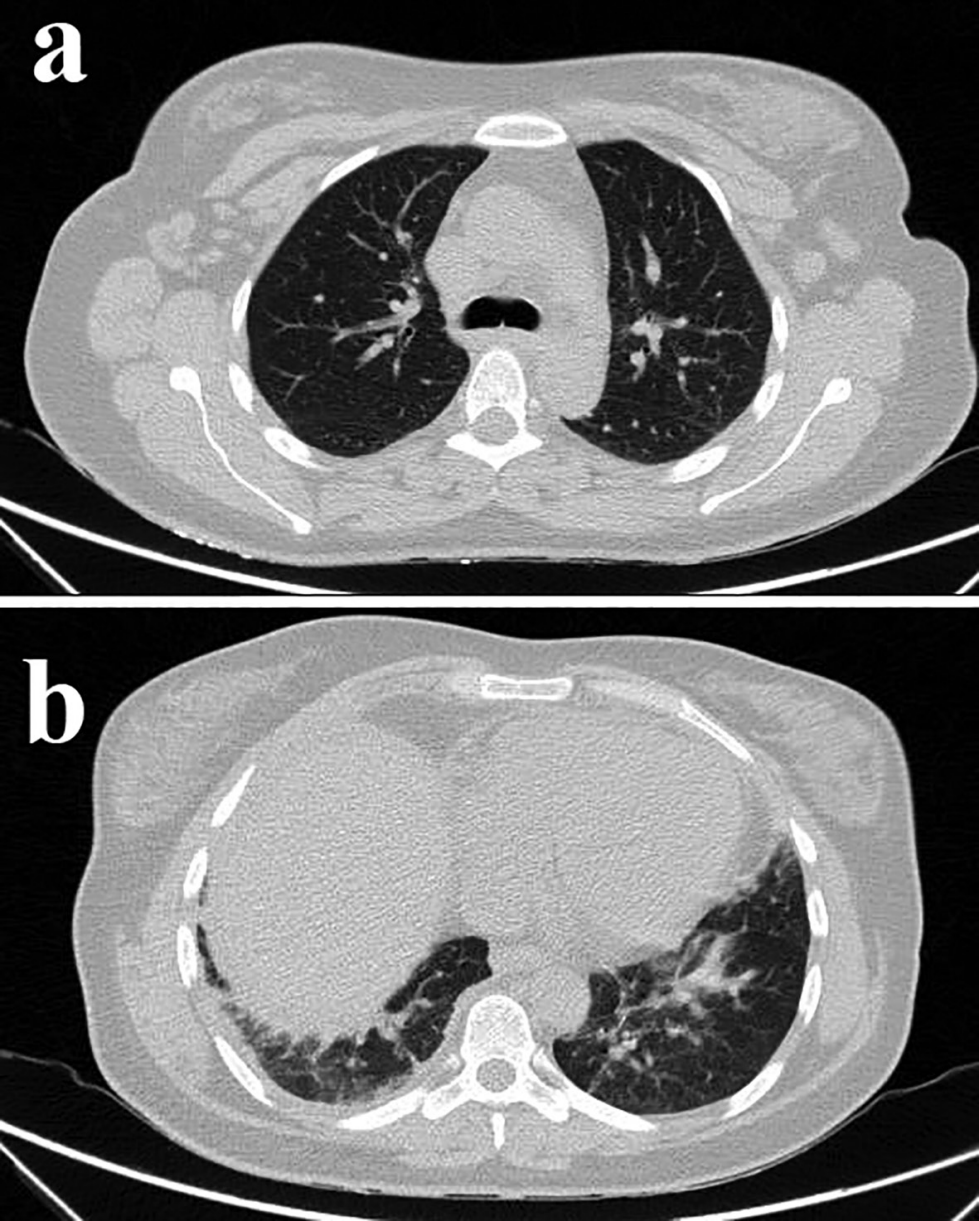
Thoracic spiral CT scan findings: (a) No evidence of active parenchymal lung disease; (b) Bilateral basal atelectasis. Note the absence of pleural or pericardial effusions.
